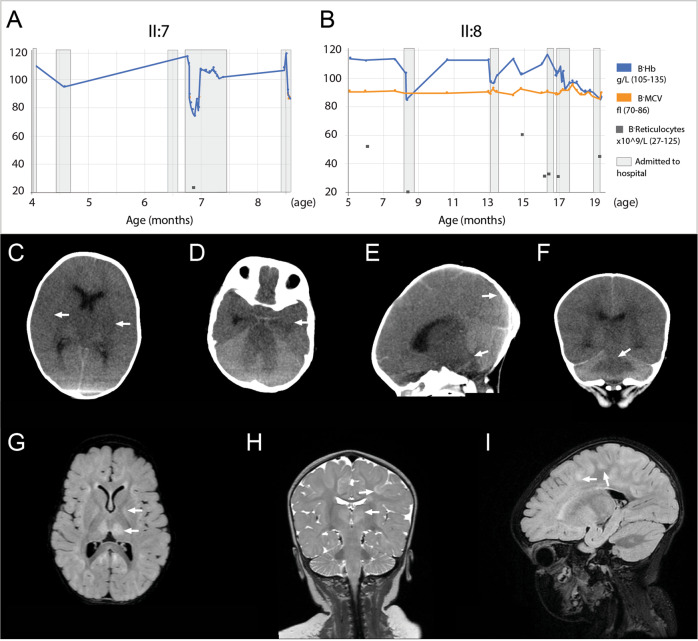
Hematological and neuroradiological investigations. A, B Both siblings developed macrocytic anemia (B-MCV > 86) with worsening during episodes of infections associated with levels of reticulocytes in the lower part of the normal level range suggestive of impaired erythropoiesis. In patient II:8, the baseline hemoglobin level tended to decrease over time. B-Hb = Blood-Hemoglobin, B-MCV = Blood-Mean Corpuscular Volume. Normal range of B-Hb, B-MCV and reticulocytes are given within parenthesis. C–F CT of the brain in patient II:7 at 8 months of age showing reduced attenuation of the entire supratentorial gray matter, compressed lateral ventricles and flattened extracerebral CSF spaces compatible with edema, loss of gray and white matter differentiation suggestive of ischemia and herniation (E, F) through the tentorium and foramen magnum. G–I In patient II:8 MRI of the brain at 8 months of age showed symmetrically increased T2 signal in thalamus, globus pallidus and in patchy cortical/subcortical fronto-parietal, paramedian and occipital areas.
